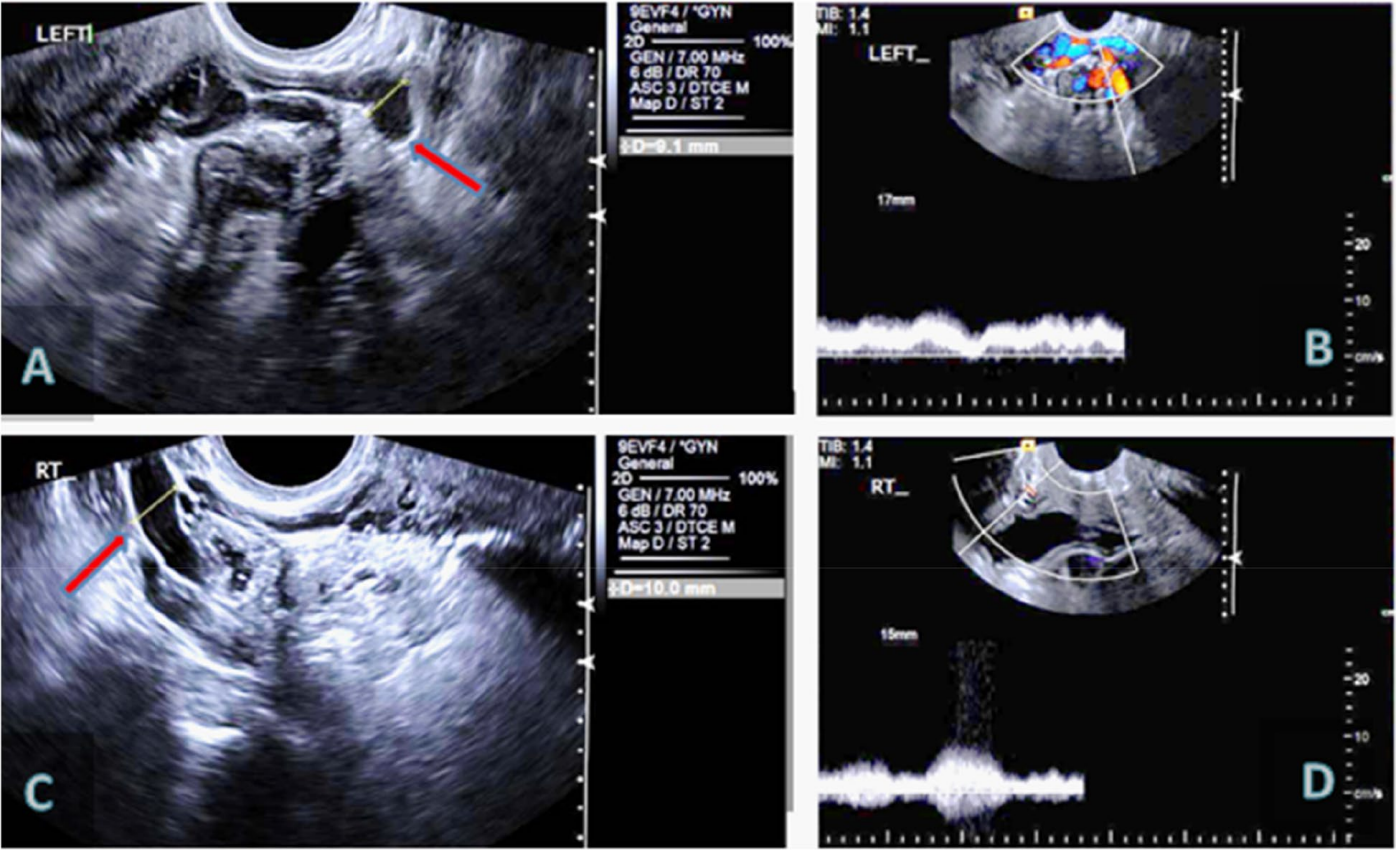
Trans-vaginal and Doppler ultrasound studies in a multiparous woman presenting with bilateral lower limb varicosities and pelvic pain. Pelvic venous congestion with left ovarian vein diameter of 9.1mm (A), and right ovarian vein diameter of 10mm (C) (red arrows), both diameters increased with Valsalva manoeuvre. Left (B) and right (D) ovarian vein Doppler studies Average flow 0.8cm/sec
